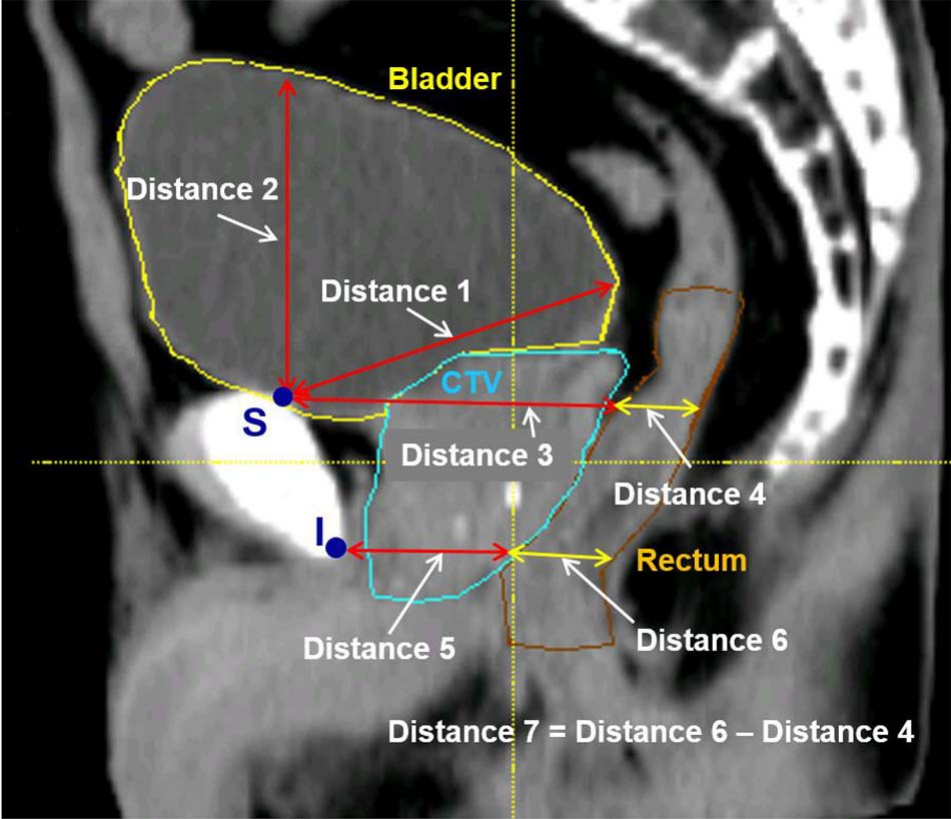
Definitions of distances 1–7 to determine the rectum and bladder anatomical features (features 1–7) selected from a planning CT image. The superior–posterior edge of the pubic bone was defined as feature point S, and the inferior–posterior edge of the pubic bone was defined as feature point I. The features express the variations of the bladder and rectum based on the pelvic bone.
